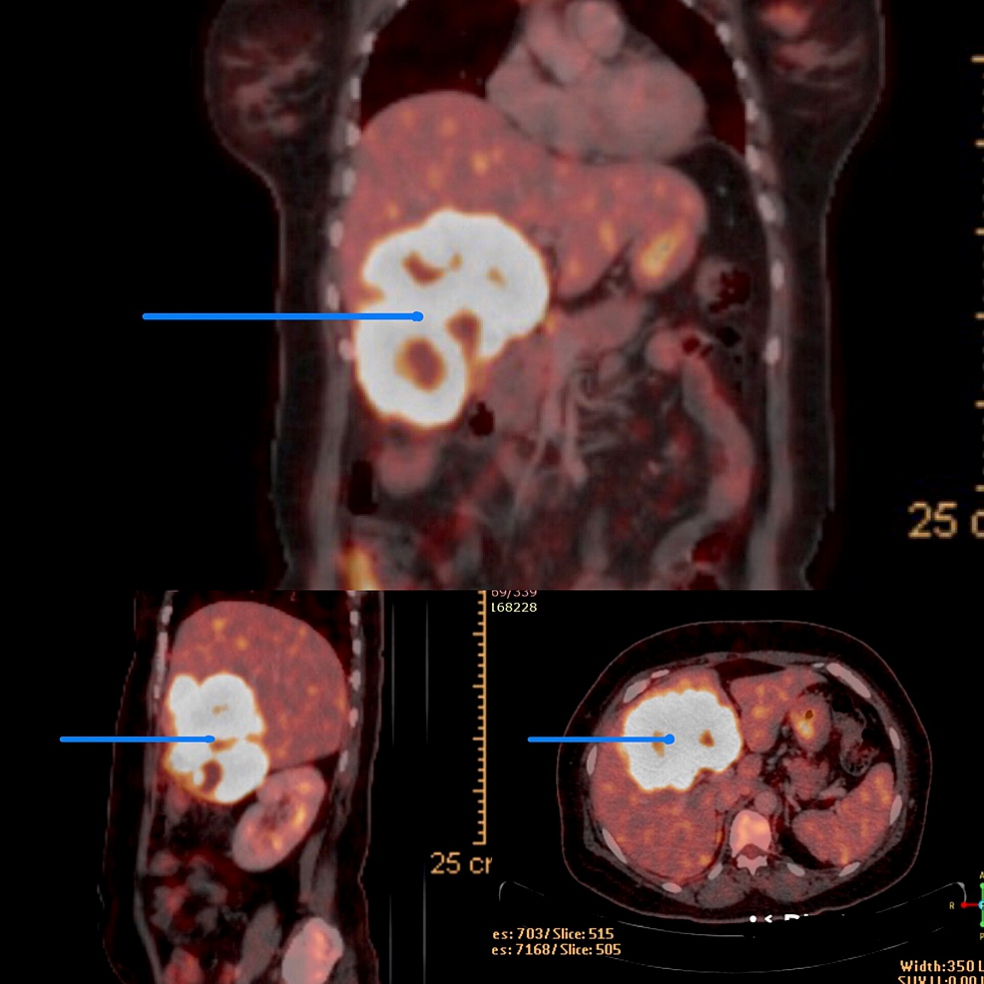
PET-CT scan showing 18-FDG wall thickening involving the gallbladder fundus with direct infiltration of the liver in the form of a large heterogeneously enhancing, partially necrotic intense 18-FDG avid with mass lesion in segment IV and V measuring approximately 9.6 x 8.4 x 10.1 cm, SUV max of 15.6 (blue arrows)
